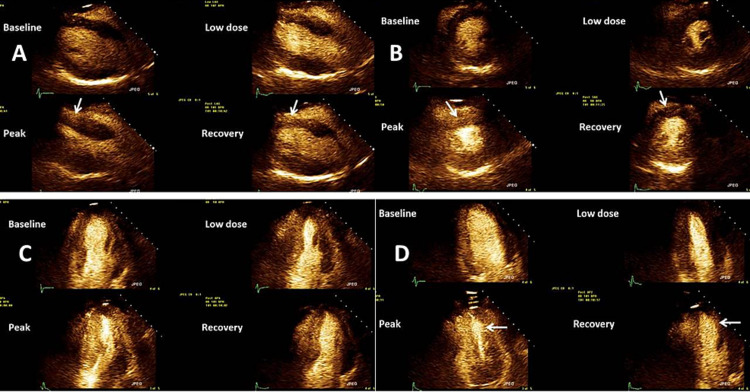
Stress echocardiogram from clinical case 3 (A) Standard parasternal long-axis views of the left ventricle showing baseline, low-dose, and peak-dose followed by recovery images. Please note that the white arrow shows a lack of contractility of the anteroseptal wall. Contractility does not improve in the recovery images. (B) Standard short-axis views of the left ventricle showing the same test phases as in the previous. Please note that the white arrow shows a lack of contractility of the anterior wall and contractility does not improve completely in the recovery images. (C) Standard four-chamber apical views of the left ventricle showing the same test phases as in the previous. (D) Standard two-chamber apical views of the left ventricle showing the same test phases as in the previous. Please note that the white arrow shows a lack of contractility of the anterior wall and contractility does not seem to improve in the recovery images
